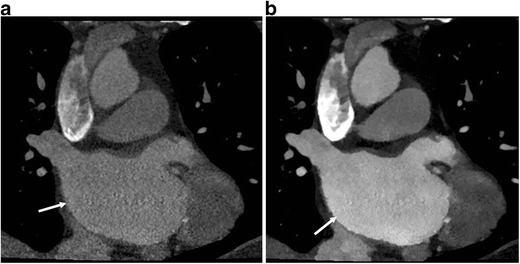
Salvage of a suboptimal vascular study. (a) Coronal 120-kVp routine diagnostic CT image in a patient being evaluated for radiofrequency ablation of pulmonary veins, shows poor contrast opacification of the vascular structures, particularly the left atrium (*arrow*), due to contrast extravasation during the scanning. (b) 45-keV virtual monoenergetic image (VMI) at the same level shows significant improvement in the contrast attenuation, especially in the left atrium (*arrow*). This obviates the need for an additional scan with another contrast bolus, saving radiation and contrast dose
